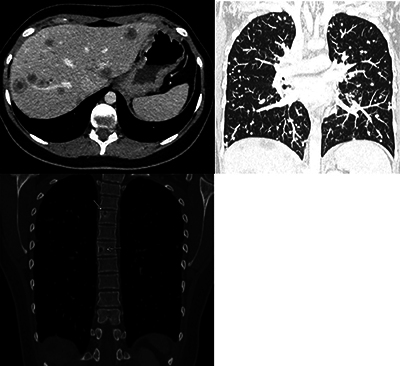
CT showing multiple, coalescent liver lesions with target appearance and peripheral enhancement (upper left), diffusely spread lung nodules (upper right) and multiple osteolytic bone lesions (lower left).
